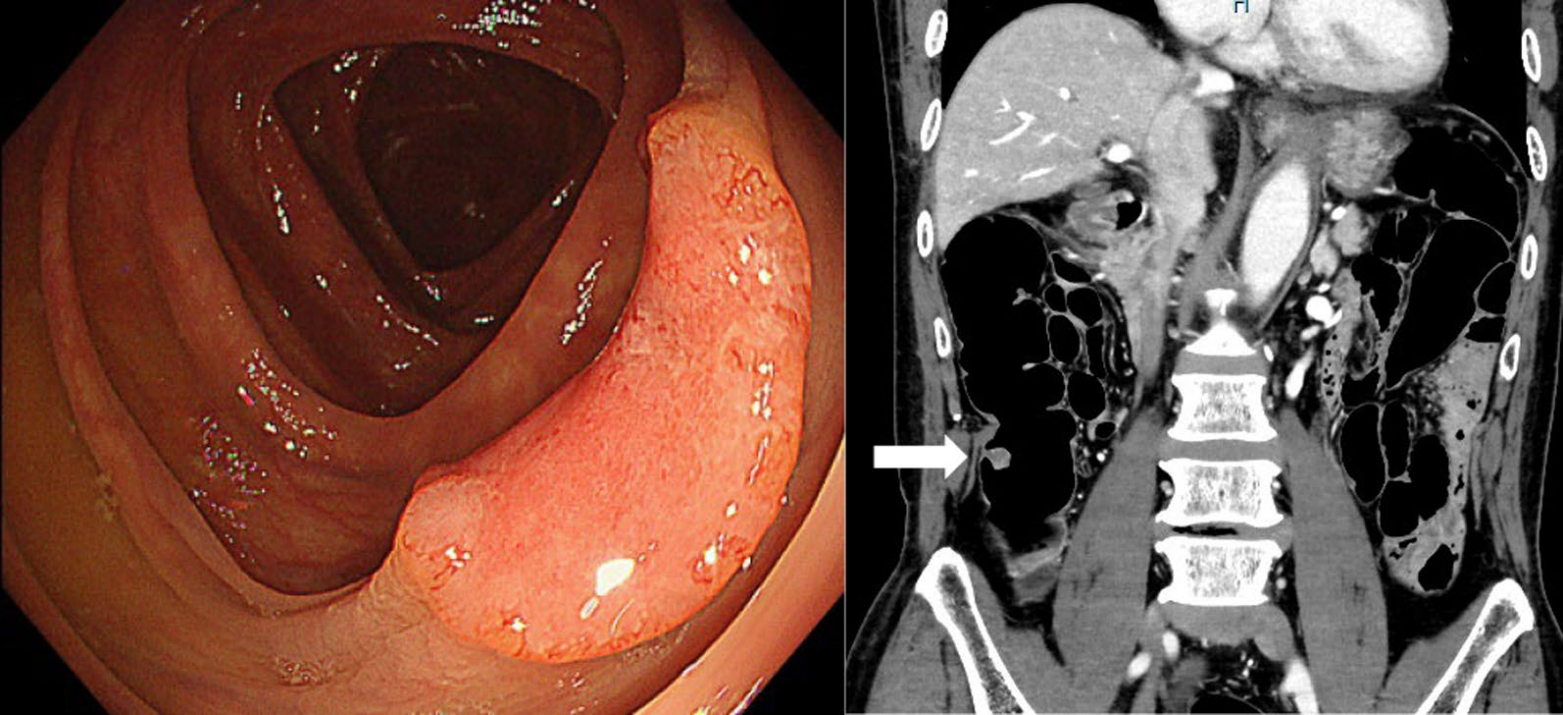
Preoperative colonoscopy examination and enhanced computed tomography (CT) scan. The colonoscopy indicates a type 2 tumor in the ascending colon, and the CT image reveals wall thickening in the ascending colon (white arrow)
